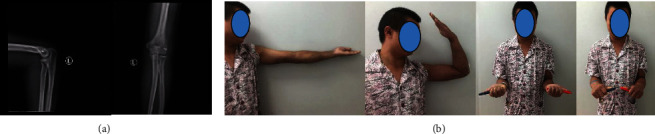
(a-1) X-ray films immediately after removal show that the elbow joint alignment is normal without dislocation. (a-2) Elbow extension function, flexion function, forearm supination function, forearm pronation function, and good stability of the elbow joint five days after removal.
